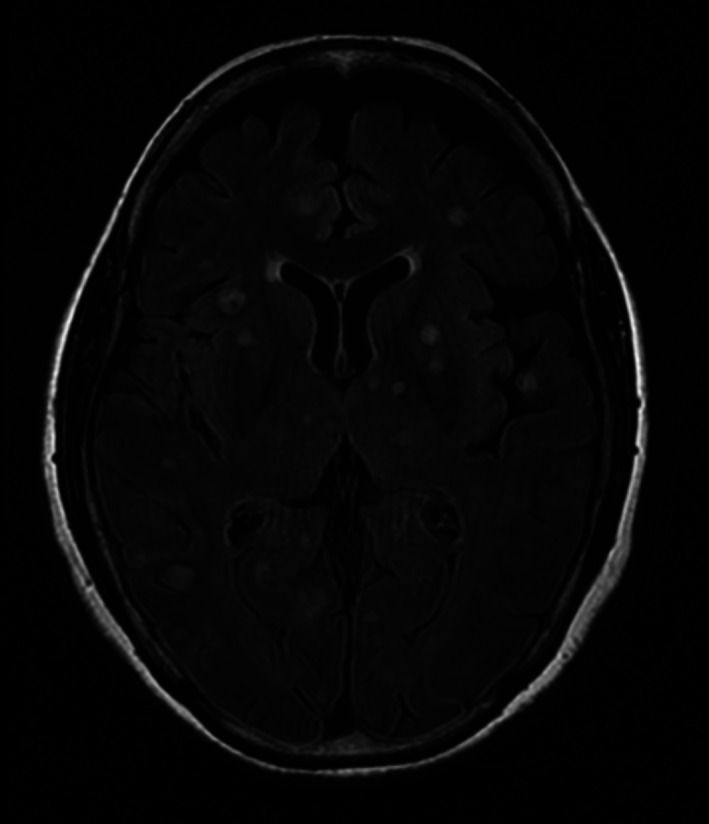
T_2_‐weighted fluid attenuated inversion recovery (FLAIR) brain magnetic resonance imaging for initial cancer status evaluation revealed multiple hyperintense lesions in the bilateral hemispheres and suspected non–small‐cell lung cancer with brain metastasis.
